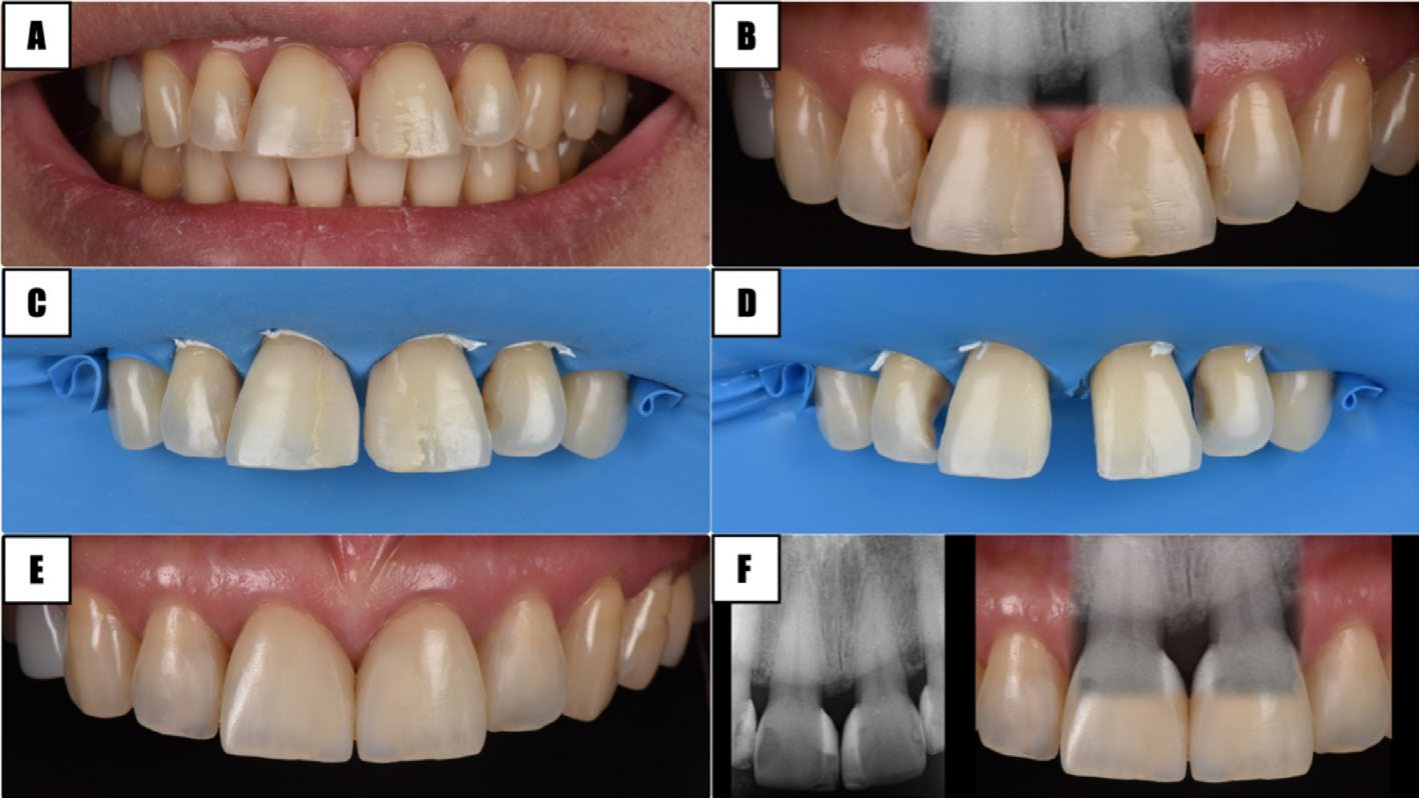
Scenario 1: healthy periodontal tissues—direct bonding. (A and B) Initial condition, (C and D) direct bonding procedure, (E and F) postoperative condition with periapical (PA) radiographs interposed.
